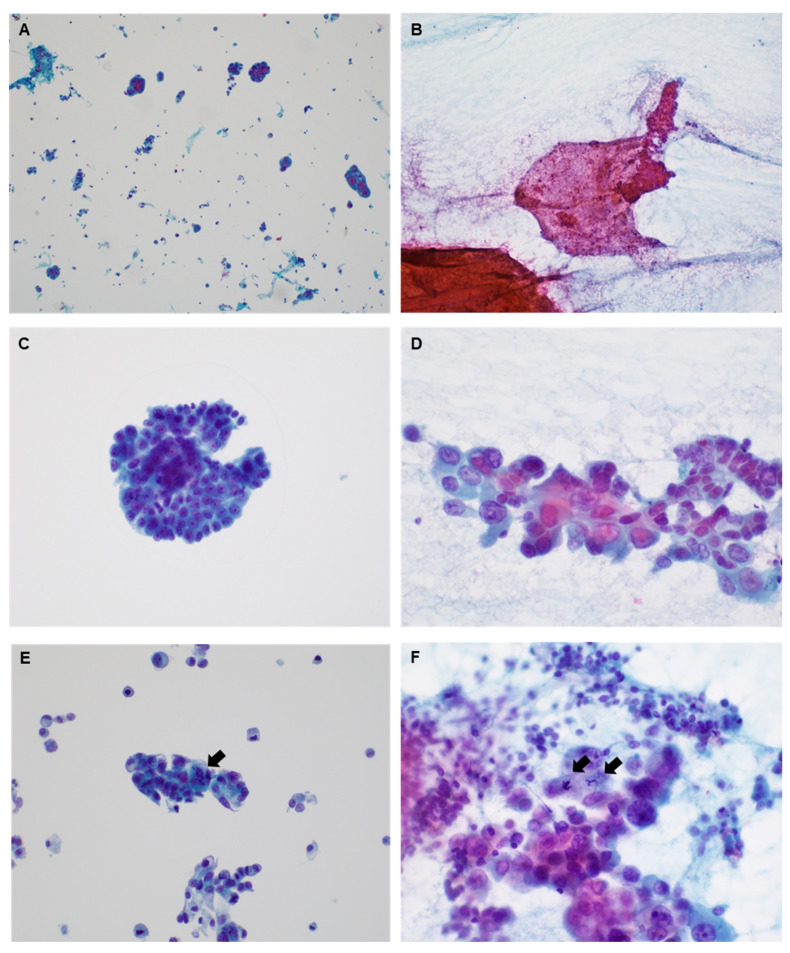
Representative images for endoscopic ultrasonography–fine needle aspiration cytology (EUS-FNAC) with liquid-based preparation (A,C,E) and conventional smear (B,D,F). (A,B) Low magnification (×100). (C–F) High magnification (×400; arrow, atypical mitosis).
